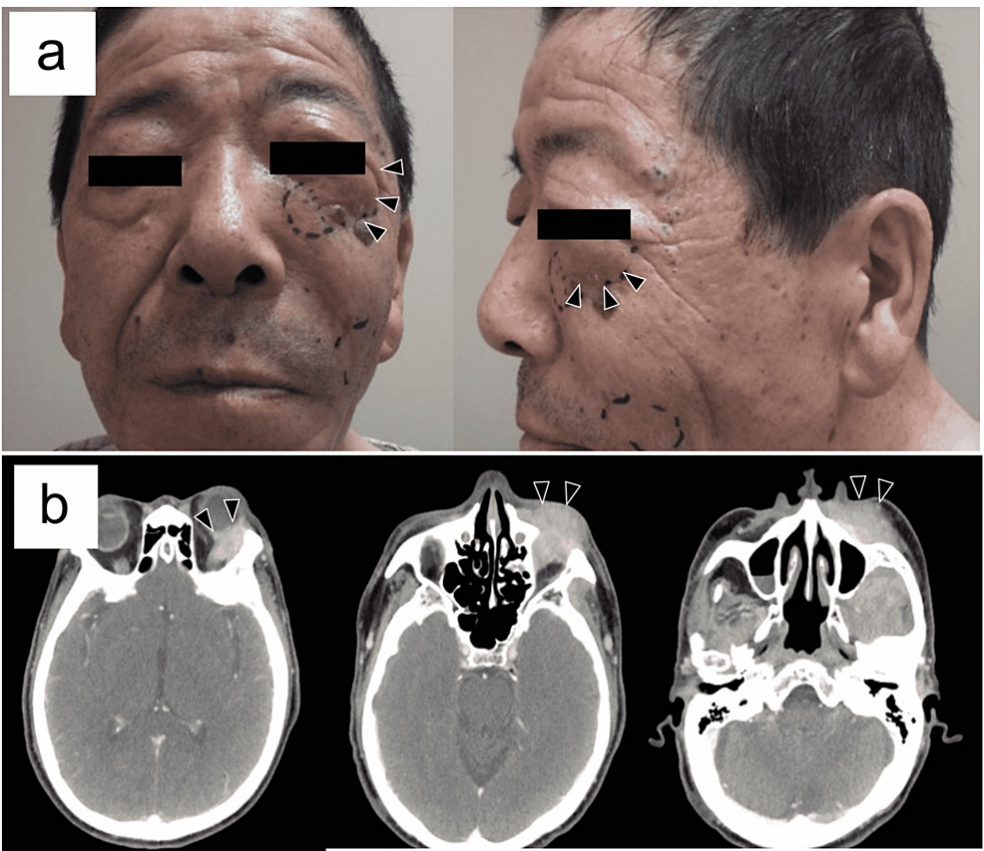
Preoperative and intraoperative findings a) Physical examination revealed swelling of the left internal canthus and cheek. Triangle lesions indicated signet-ring cell/histiocytoid carcinoma. b) Computed tomography showed an ill-defined mass in the left orbit, buccal skin, masticator space, and maxilla (arrowheads).
